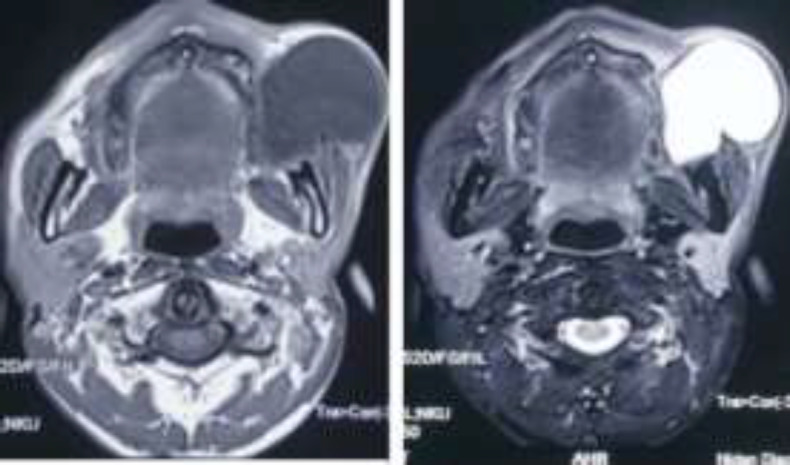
Contrast magnetic resonance imaging (T1 and T2 weighted) showing a thick-wall cystic swelling in the left buccal space over the temporalis muscle
